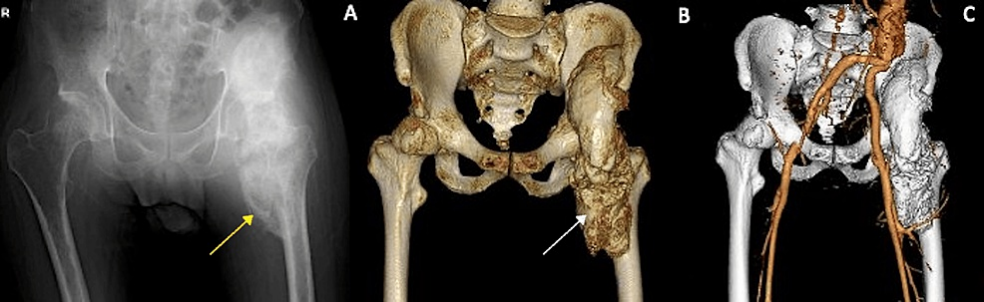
Patient 1 preoperative images Patient 1 preoperative X-ray (A), CT scan of the hip (B), and CT angiogram reconstruction of the vasculature (C). The yellow arrow depicts heterotopic ossification visible on X-ray. The white arrow shows the extent of heterotopic ossification on the CT scan. CT: computed tomography
